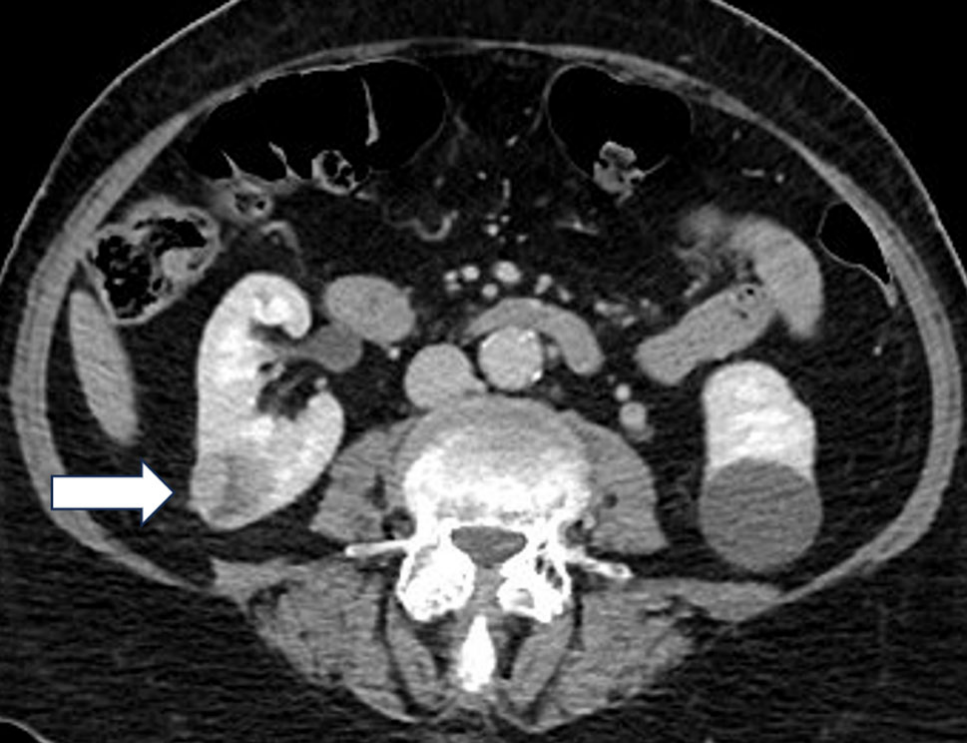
Axial contrast-enhanced CT showed an enhancing right lower pole RCC measuring 2.81 cm (maximum diameter, core lab assessed)
